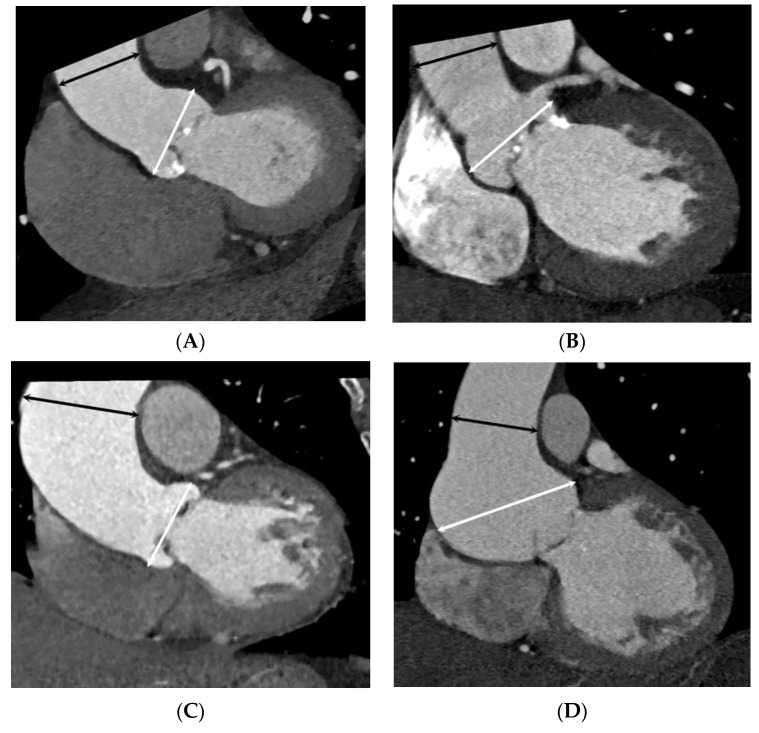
Diameters of the ascending aorta measured via cardiac computed tomography at two different levels (sinus of Valsalva [white arrow] and tubular portion [black arrow]) at mid-to-end systole. The ascending aorta was assigned to one of four main anatomical types according to the segment of the vessel exclusively or predominantly involved in dilatation based on the indexed maximal aorta diameter of exceeding 21 mm/m^2^ of body surface area: (A) normal; (B) type 1, root dilatation; (C) type 2, ascending aorta dilatation; (D) type 3, combined dilatation of the aortic root and middle ascending aorta.
